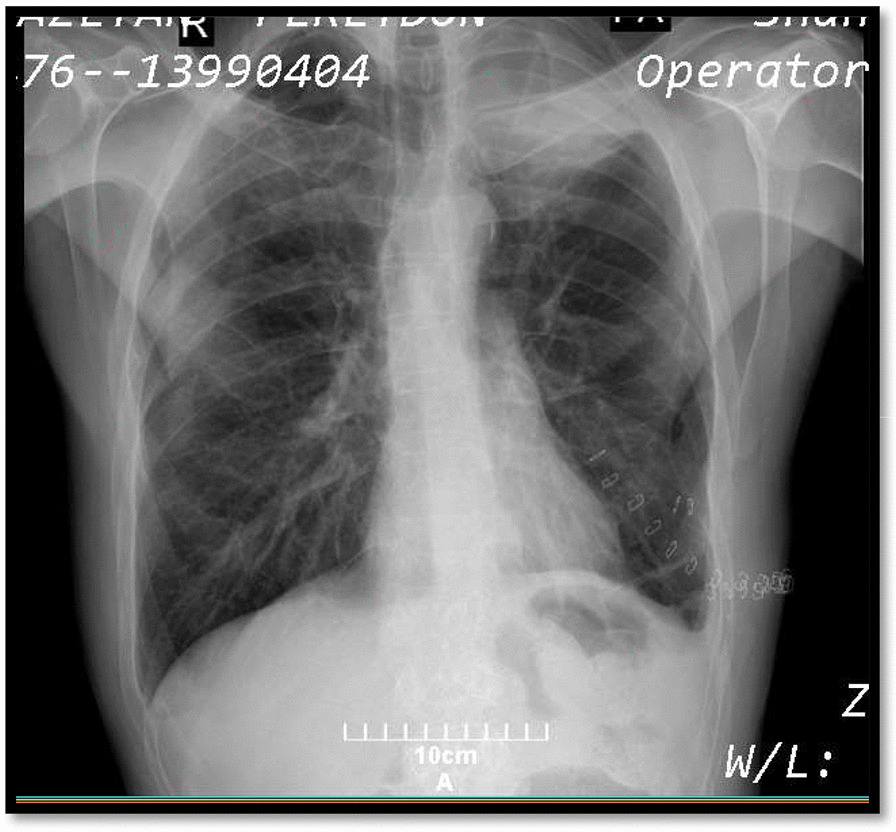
Chest radiography (2nd admission), at the time of discharge from the hospital showing discontinued chest tube, and resolved pneumothorax
